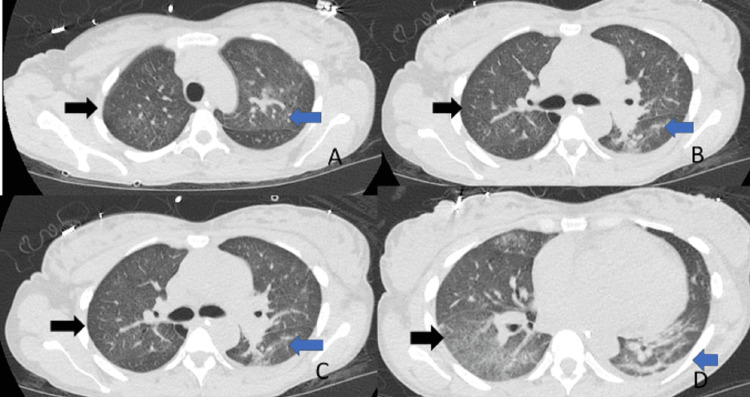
A follow-up CT of the chest after eight days of chest tube placement Lung windows, resolution of right pleural effusion (black arrows), and residual pulmonary infiltrates (blue arrows). A. upper lobes; B. carina; C. middle lobe; D. lower lobes
